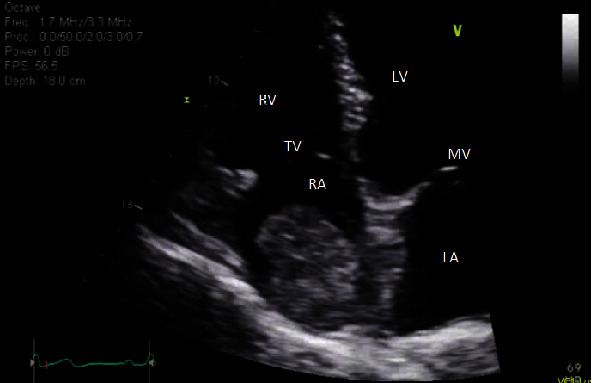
Transthoracic echocardiogram, four chamber view. Mass depicted in the right atrium, not obstructing the tricuspid valve. RV = right ventricle; LV = left ventricle; RA = right atrium; LA = left atrium; MV = mitral valve; TV = tricuspid valve.
